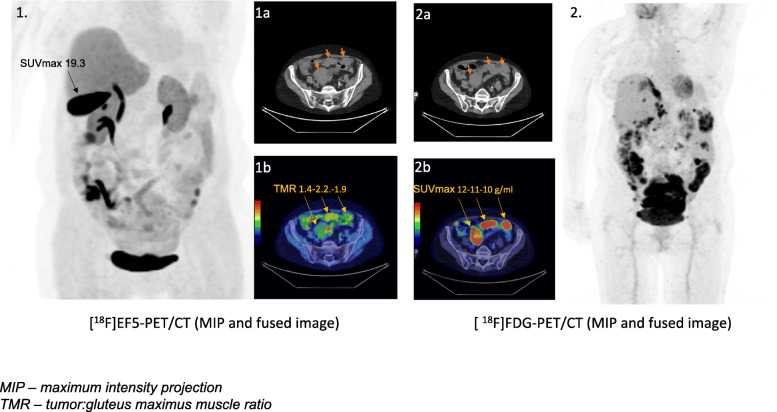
An image of a patient with advanced ovarian cancer. All three metastases are FDG-avid (2b) and 2 of them have also accumulated EF5 (1b). The SUVmax-values in [^18^F]EF5-avid (tumor:gluteus maximus muscle ratio ≥ 1.5) are remarkably lower (1b) compared to the physiological uptake in bile (1.)
